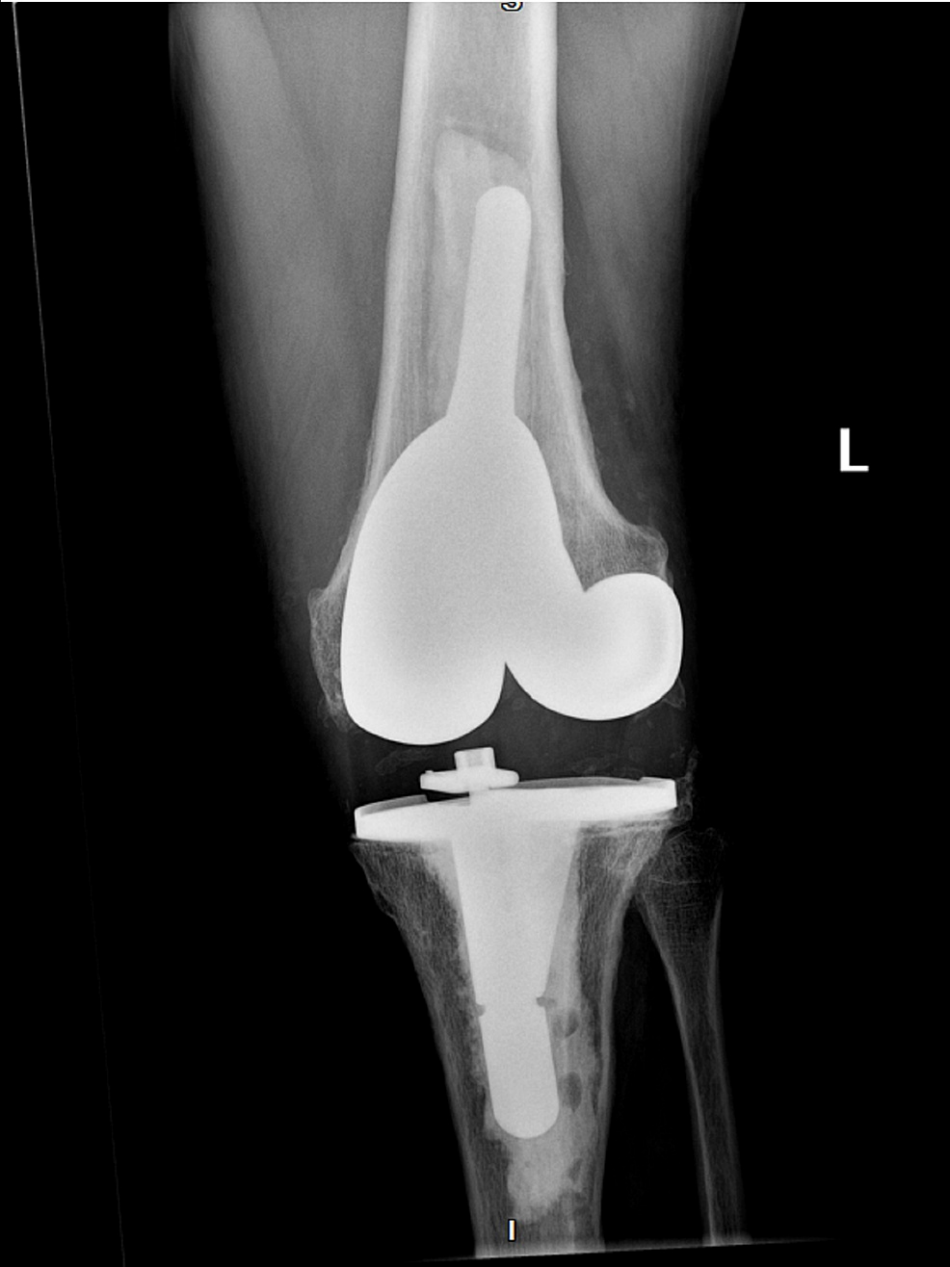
X-ray of the infected left knee with total arthroplasty prior to explantation (anteroposterior)
